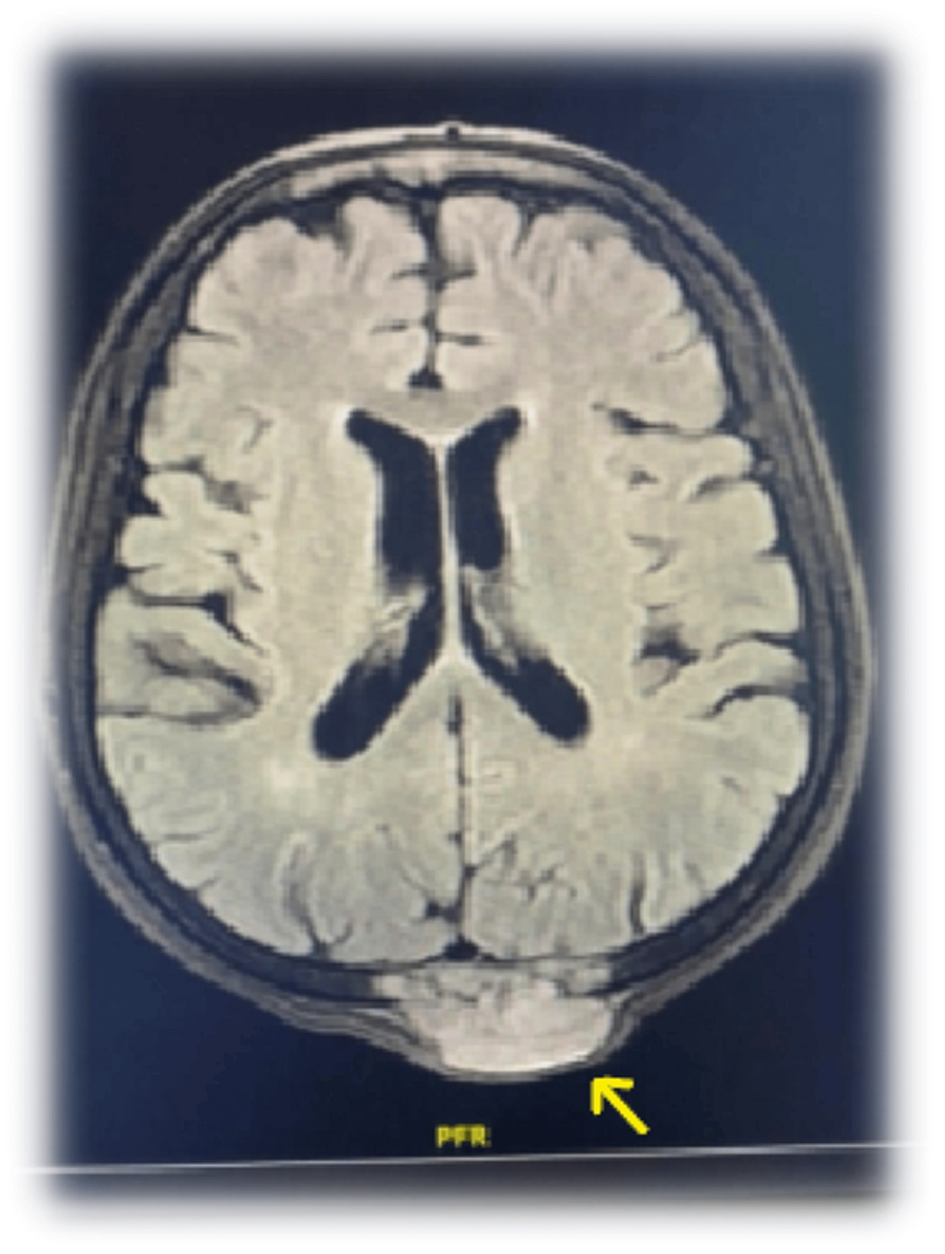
A 73-year-old female patient with oncocytic cancer exhibiting cranium metastasis, as evidenced by magnetic resonance imaging (MRI).
